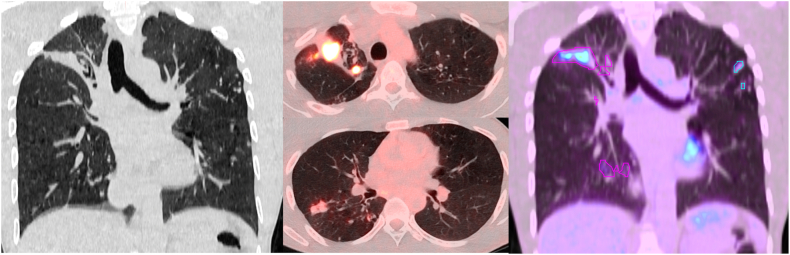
Computed tomography (CT) and 18F-FDG-PET/CT scans of a 33-year-old male from the Gambia with previous history of tuberculosis who presented with epigastric pain, weight loss and fatigue. The CT scan revealed a consolidation in the right upper lobe consistent with post-tuberculosis lung disease such as fibrotic bands (left image, coronal CT scan). The PET/CT shows FDG-avid hot lesions (SUVmax 9.06 SUVbw) in the right upper lobe (top middle picture) and a smaller consolidation in the middle lobe (SUVmax 2.69 SUVbw, bottom middle picture). Total lung glycolysis (TLG) is a semiautomated technique to calculate the total lung glycolytic activity above a threshold (background lung avidity above SUVmax = 1, right picture). Highlighted regions of interest (ROIs) are circulated in pink and light blue. (For interpretation of the references to colour in this figure legend, the reader is referred to the Web version of this article.)
